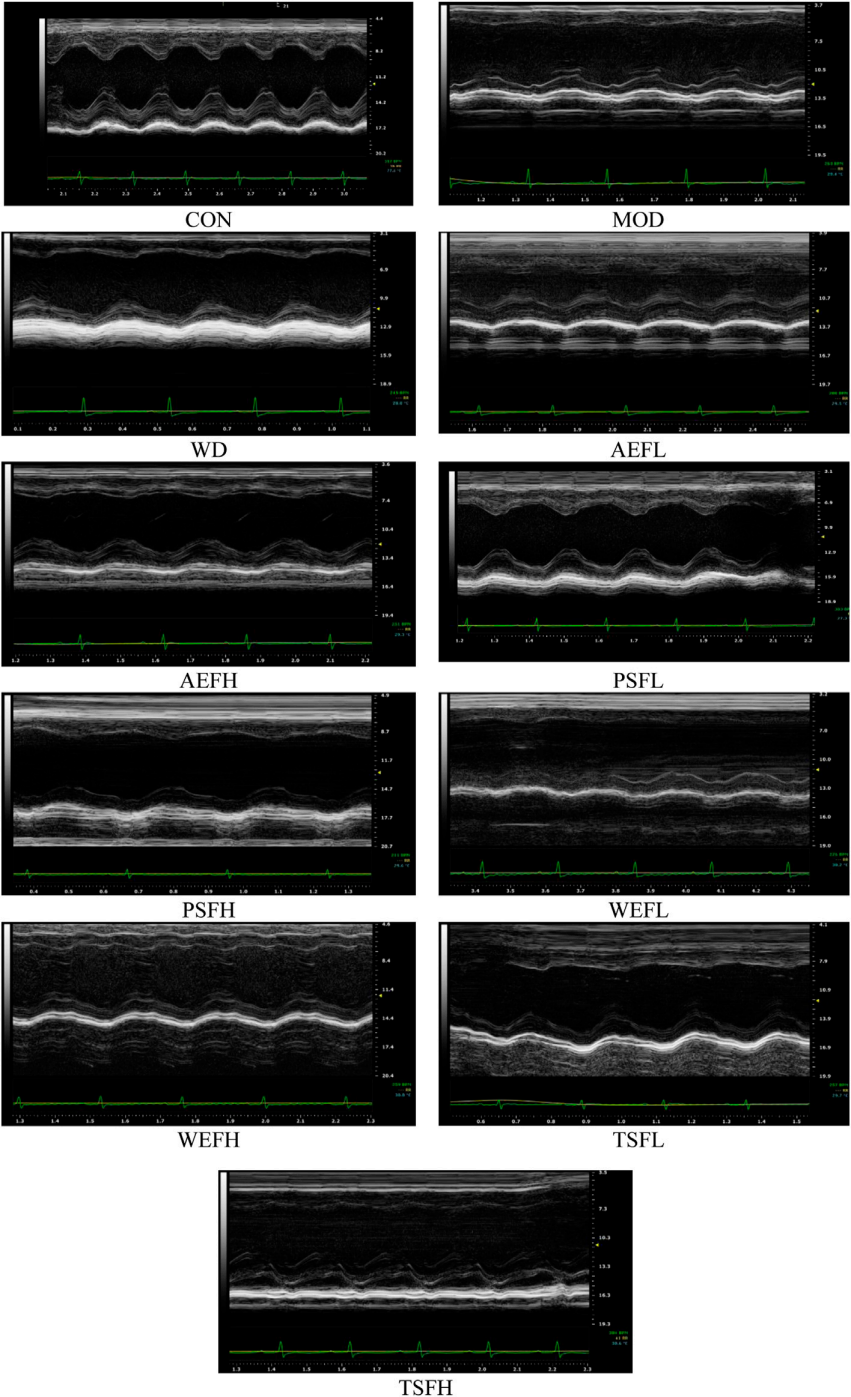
Effects of BG and its fractions on echocardiographic examinations.
